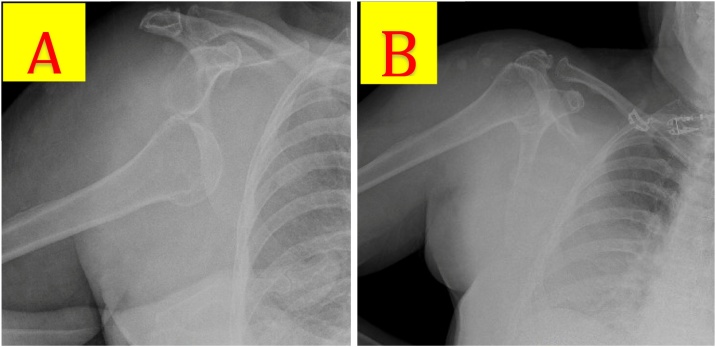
A) Index right shoulder radiograph: Inferior glenohumeral dislocation with greater humeral tuberosity abutting the infraglenoid tubercle. B) Post-reduction radiograph with no acute fractures or deformities.
